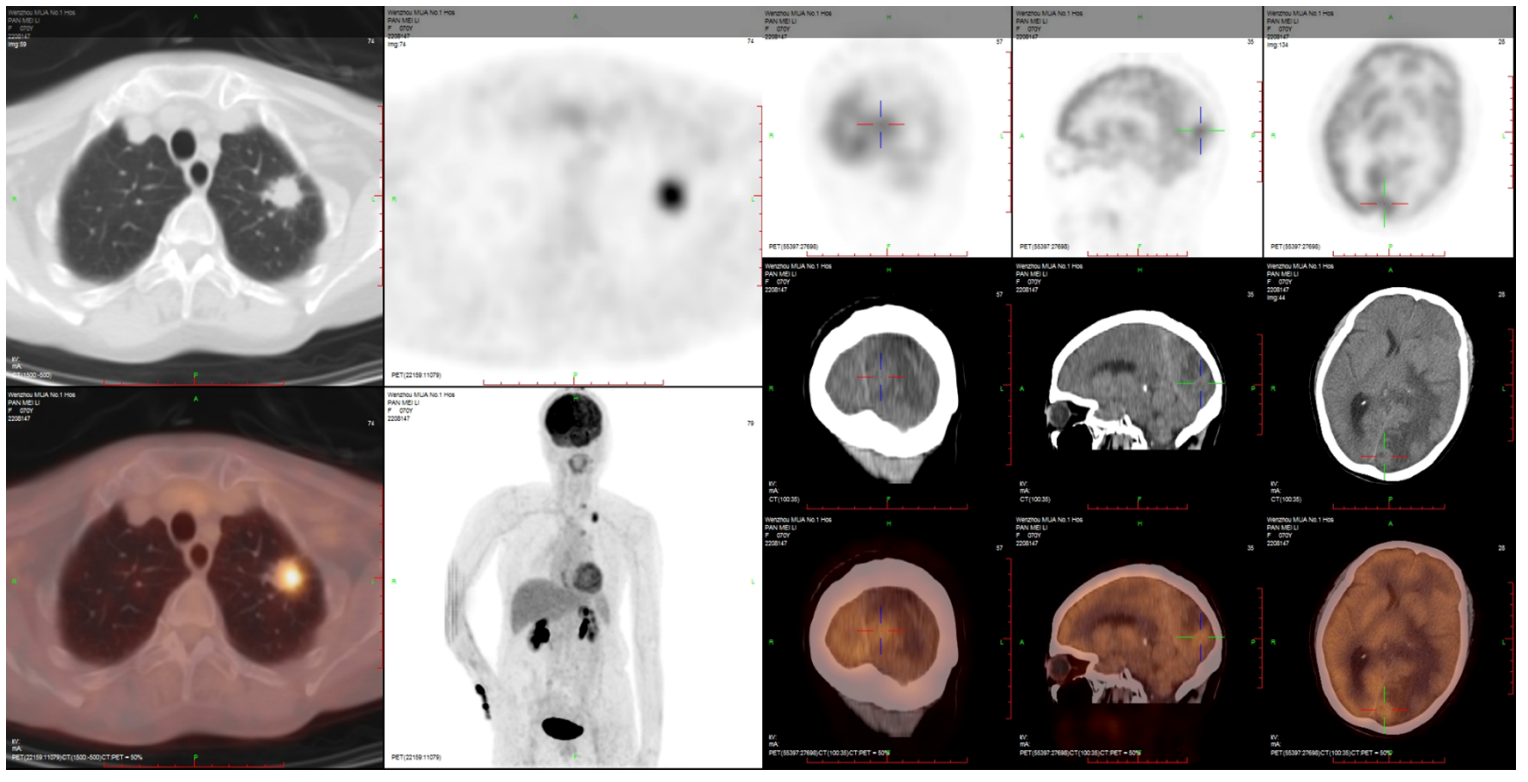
PET-CT on 10 April 2022.
